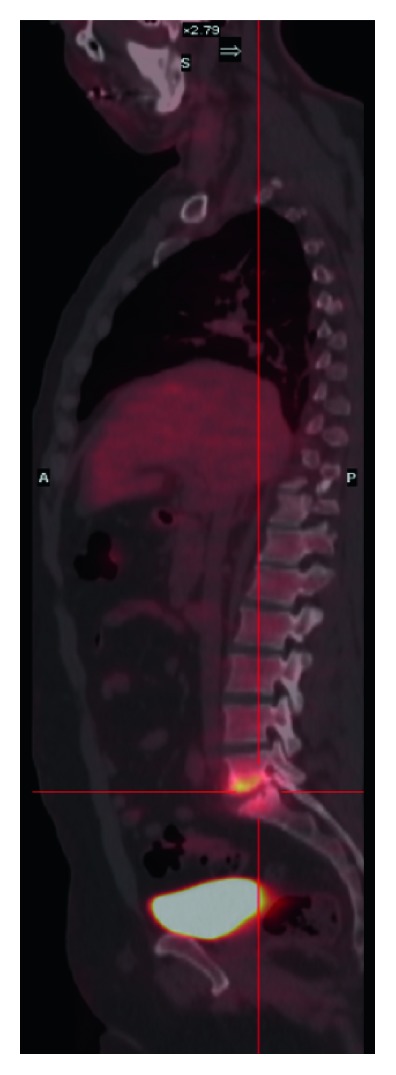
FDG-PET/CT scan of patient 2 showing an intense metabolic uptake between the lower plate of L5 and the upper plate of S1.
